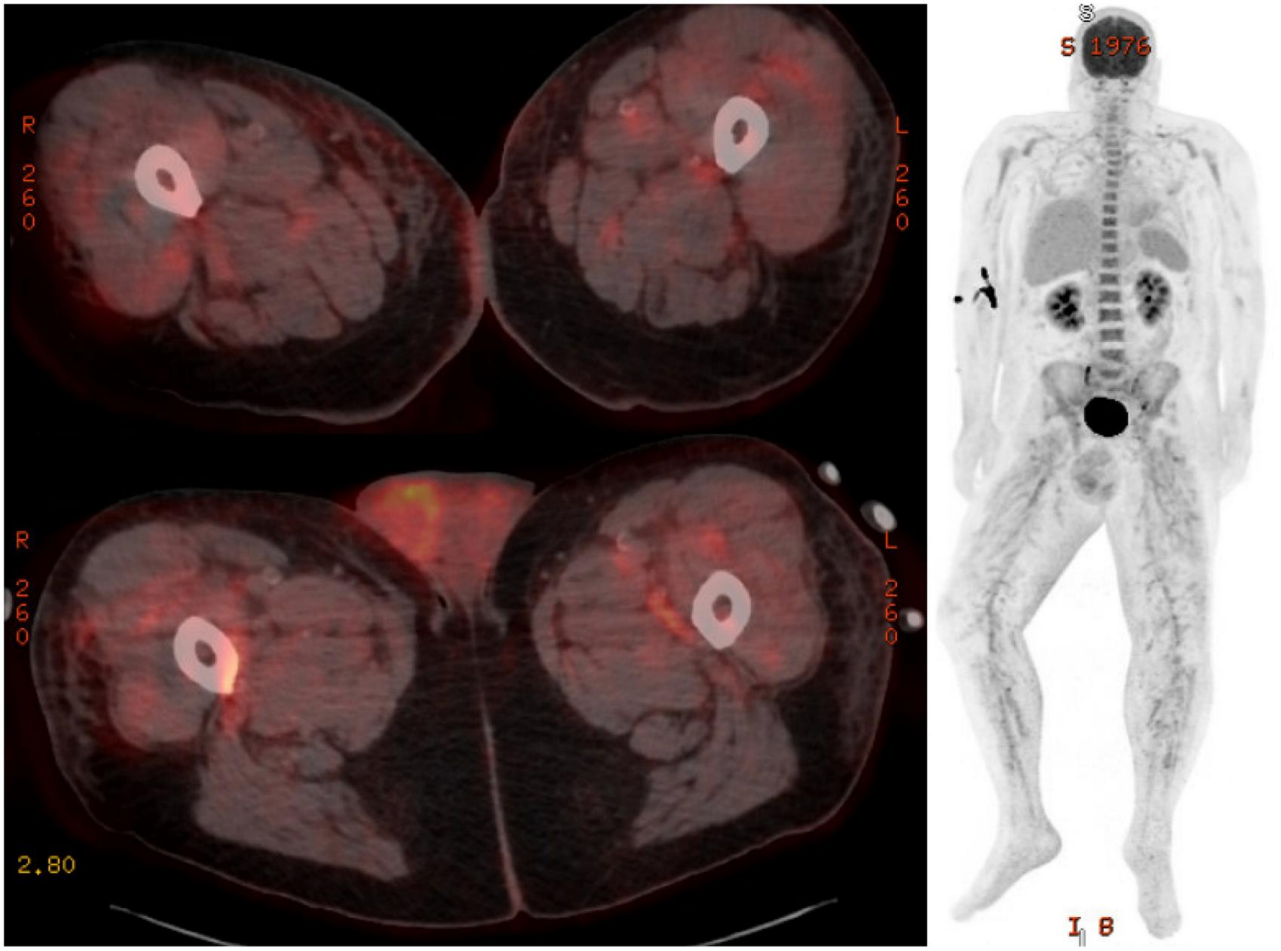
F-18 FDG PET CT scan with axial imaging of the scrotum and proximal thighs (left), as well as maximum intensity projection image (right). Demonstrates diffuse hypermetabolic FDG activity associated predominantly with medium to small arterial vasculature throughout the whole body (right). This is most pronounced in the bilateral lower extremities, with additional enhancement in the bilateral supraclavicular and axillary regions, and pelvis/scrotum.
